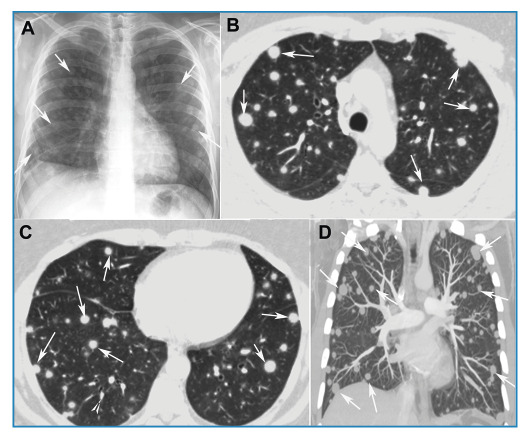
(A) Anteroposterior chest radiograph demonstrating multiple nodules of varying sizes in both lungs (arrows). Axial chest computed tomography images (B) and (C) and a coronal maximum-intensity projection reconstruction (D) showing multiple homogeneous, sharply circumscribed, randomly distributed nodules in both lungs (arrows).
